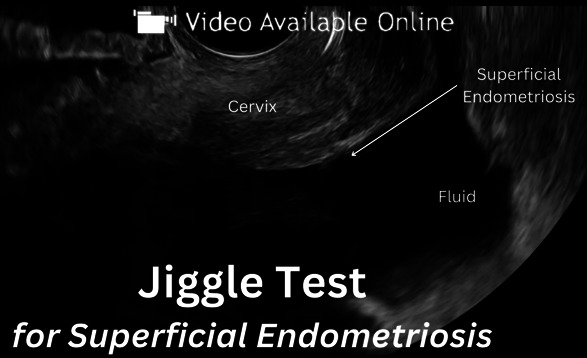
Video 1. Demonstration of the jiggle test for detecting superficial endometriosis. This technique involves applying gentle pressure with the transvaginal ultrasonography probe to induce movement of pelvic fluid, allowing visualization of superficial endometriosis lesions along the peritoneal surface. Video created by Ido Mick, Shay M. Freger, Melissa Marien, Mahsa Gholiof, and Mathew Leonardi. Used with permission.
